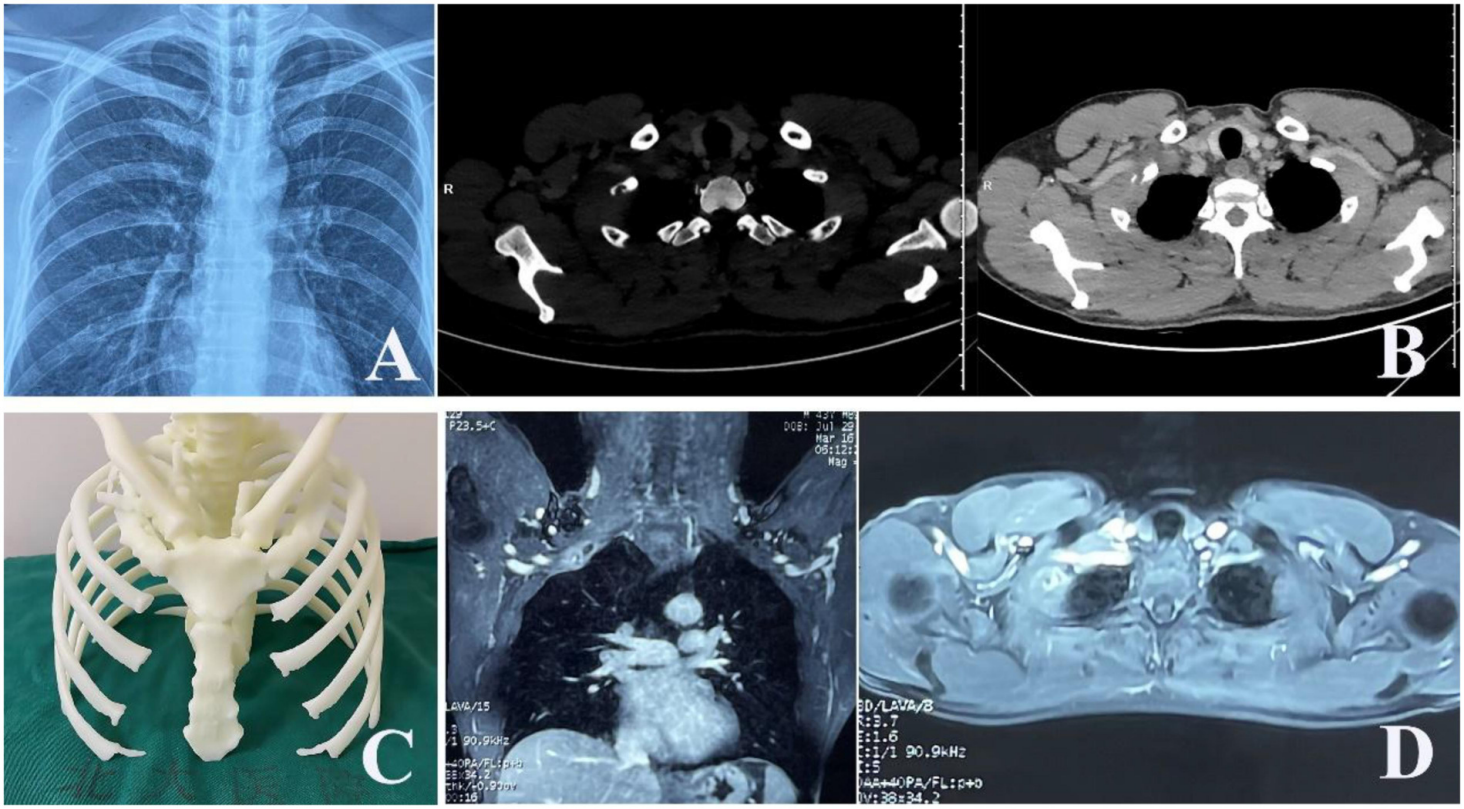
(A) An X-ray shows a lytic lesion and fracture in the right first rib; (B) a CT scan shows osteolytic destruction in the mid-segment of the right first rib, with soft tissue swelling; (C) the 3D printed model based on the CT scan; (D) T2-weighted MRI images show high-intensity signals in the middle part of right first rib.
